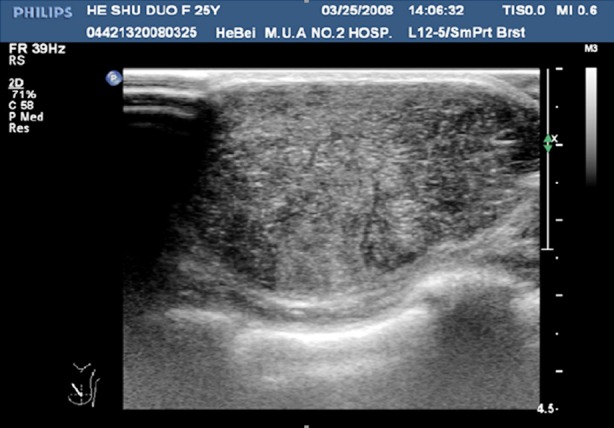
Ultrasonography of benign fibroadenoma.
